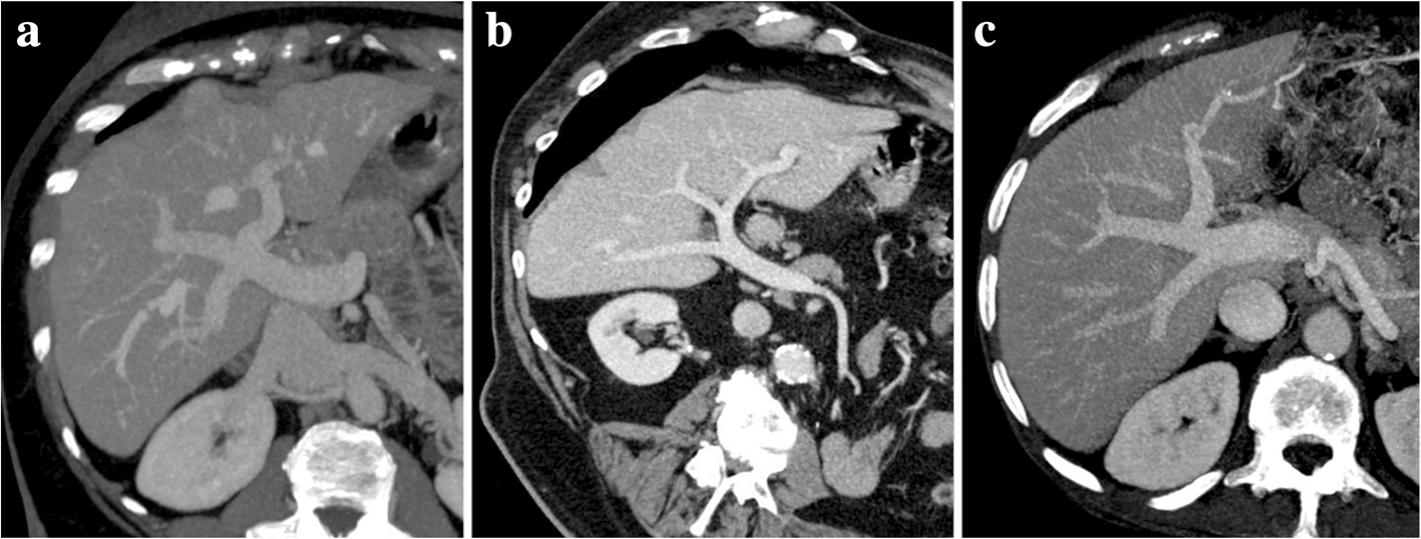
Variants of the PV branching pattern. MIP oblique reconstruction images from portal venous phase CT. a PV trifurcation—PV divides into three branches: left PV, right anterior PV, and right posterior PV. b Right posterior PV branch arising directly from the main PV. c Left PV arising from the right anterior segmental branch
